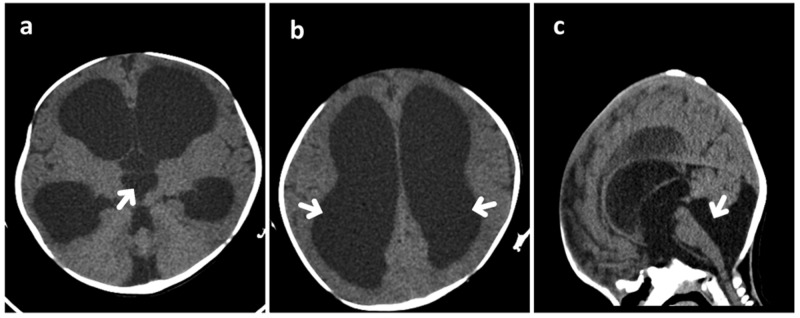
Non-enhanced computed tomography images (NECT) Axial (a,b) and sagittal (c) scans showed marked dilation of the lateral and third ventricles, mildly dilated fourth ventricle, stretching of corpus callosum, and bulging and widening of the anterior fontanelle. The cerebral aqueduct was open and communicated with the cisterna magna. Prominent infravermian cerebrospinal fluid space; however, there was a normally developed cerebellar vermis.
